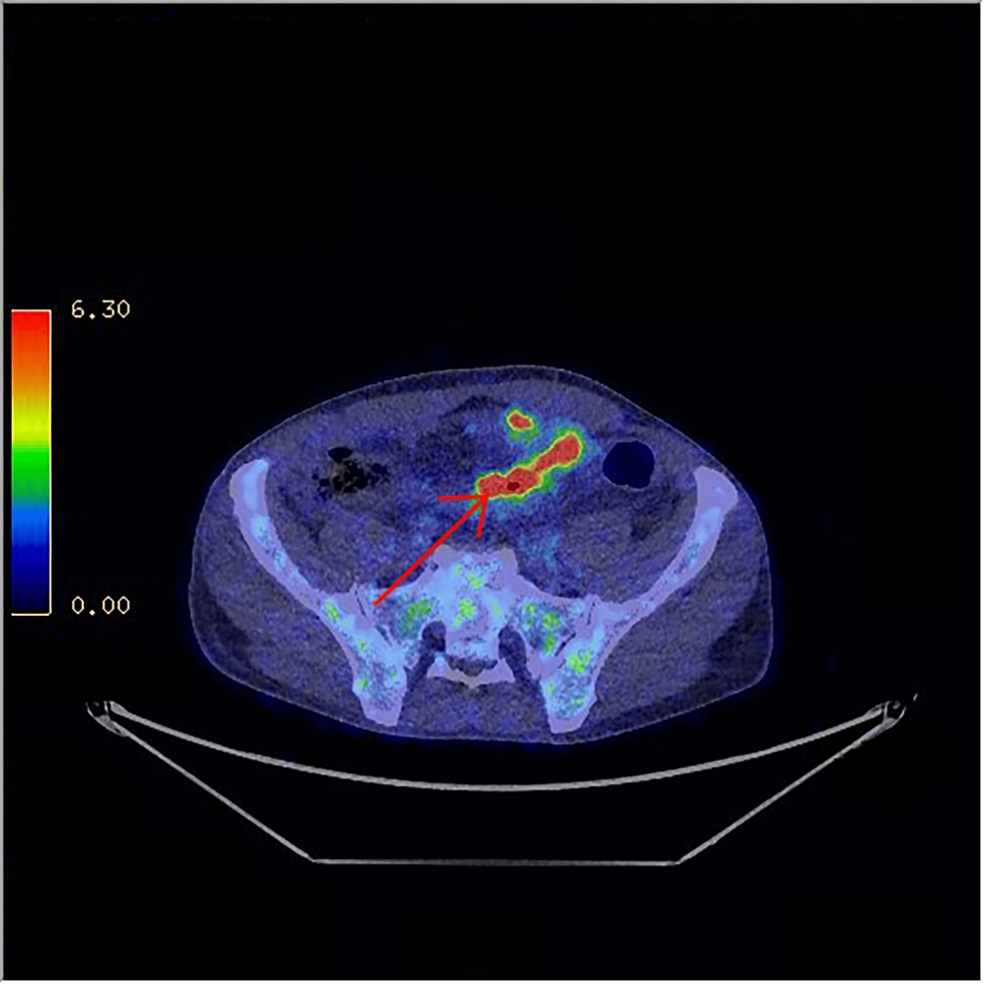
PET CT scan showing a persistent hypermetabolic focus in the sigmoid colon after one month of discharge from the hospital. PET, positron emission tomography; CT, computed tomography
